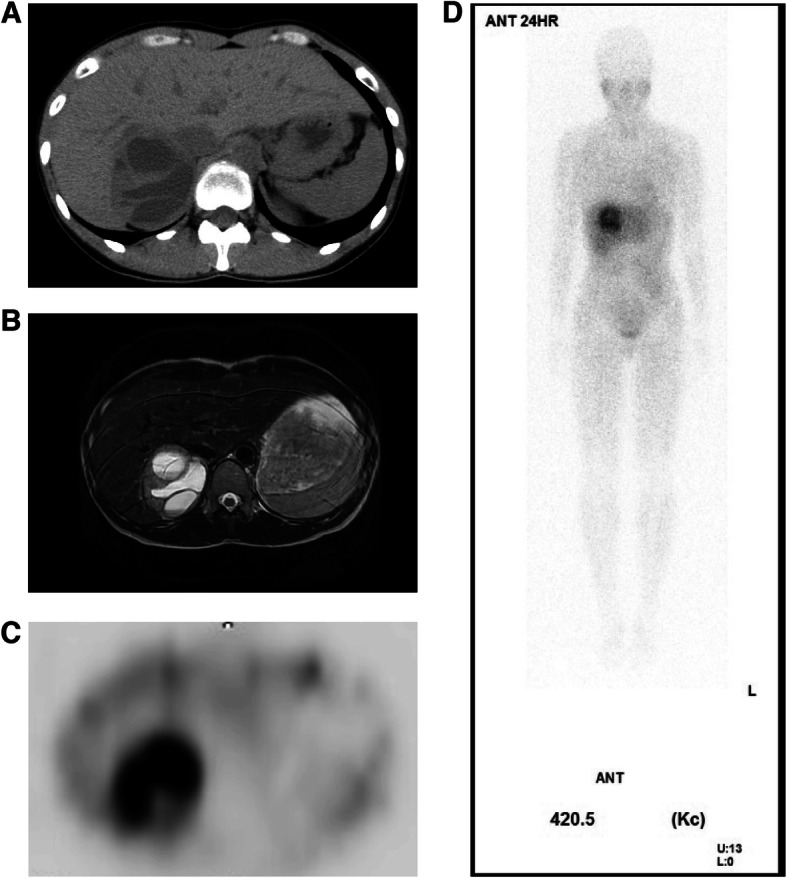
a Abdominal computed tomography (CT) shows a mass with cystic components in the right adrenal gland. b MRI shows modestly high intensity with cystic components in T2-weighted image. c and d In ^123^I-MIBG scintigram, increased trace uptake was observed in the right adrenal gland mass
